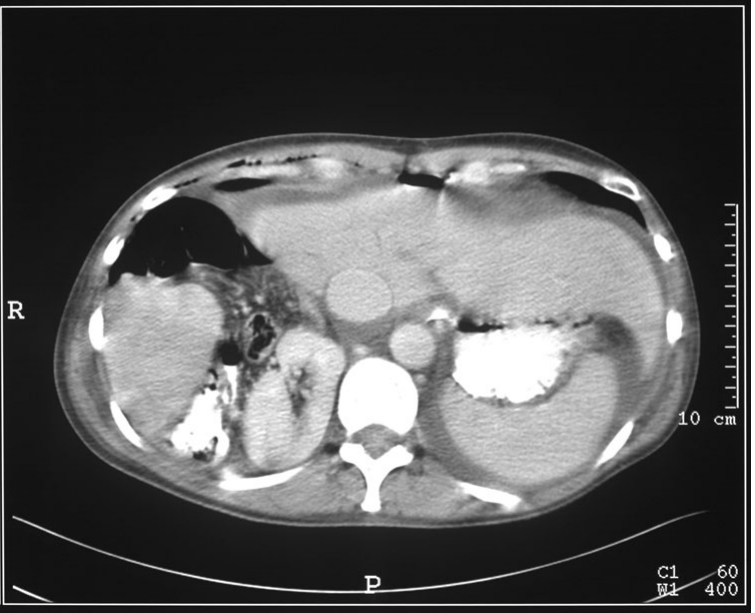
CT scan of thorax and abdomen demonstrating the abnormal anatomy
